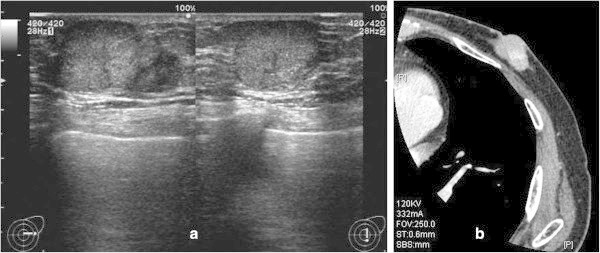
Ultrasonography (a) and computed tomography (b) revealed a mass in the left breast with no enlargement of either axillary or cervical lymph nodes.
